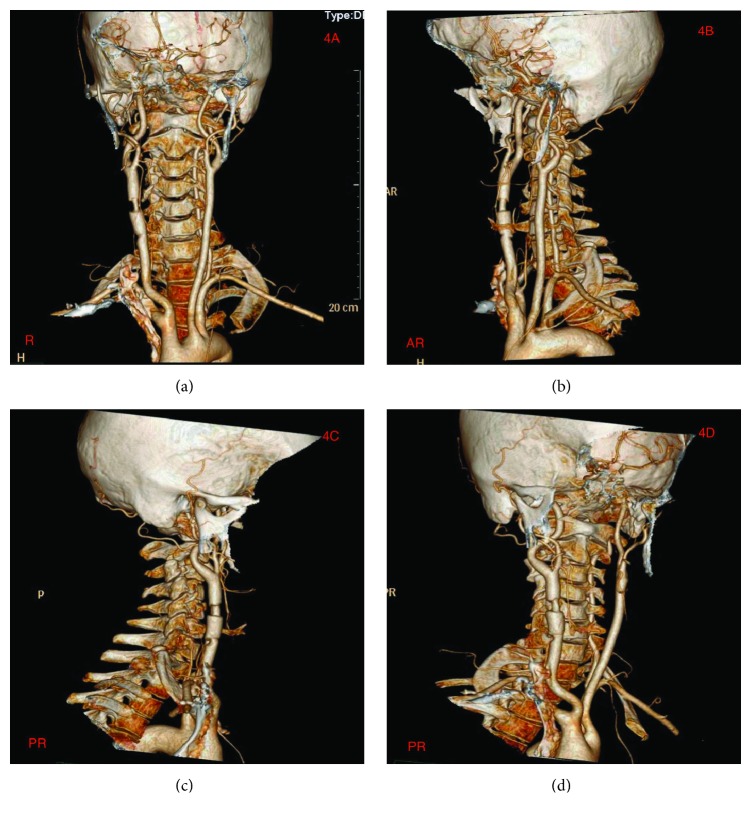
(a)-(d) are CTA showing the fractures at spinous processes of C2-C4 as well as right common carotid artery dissection.
